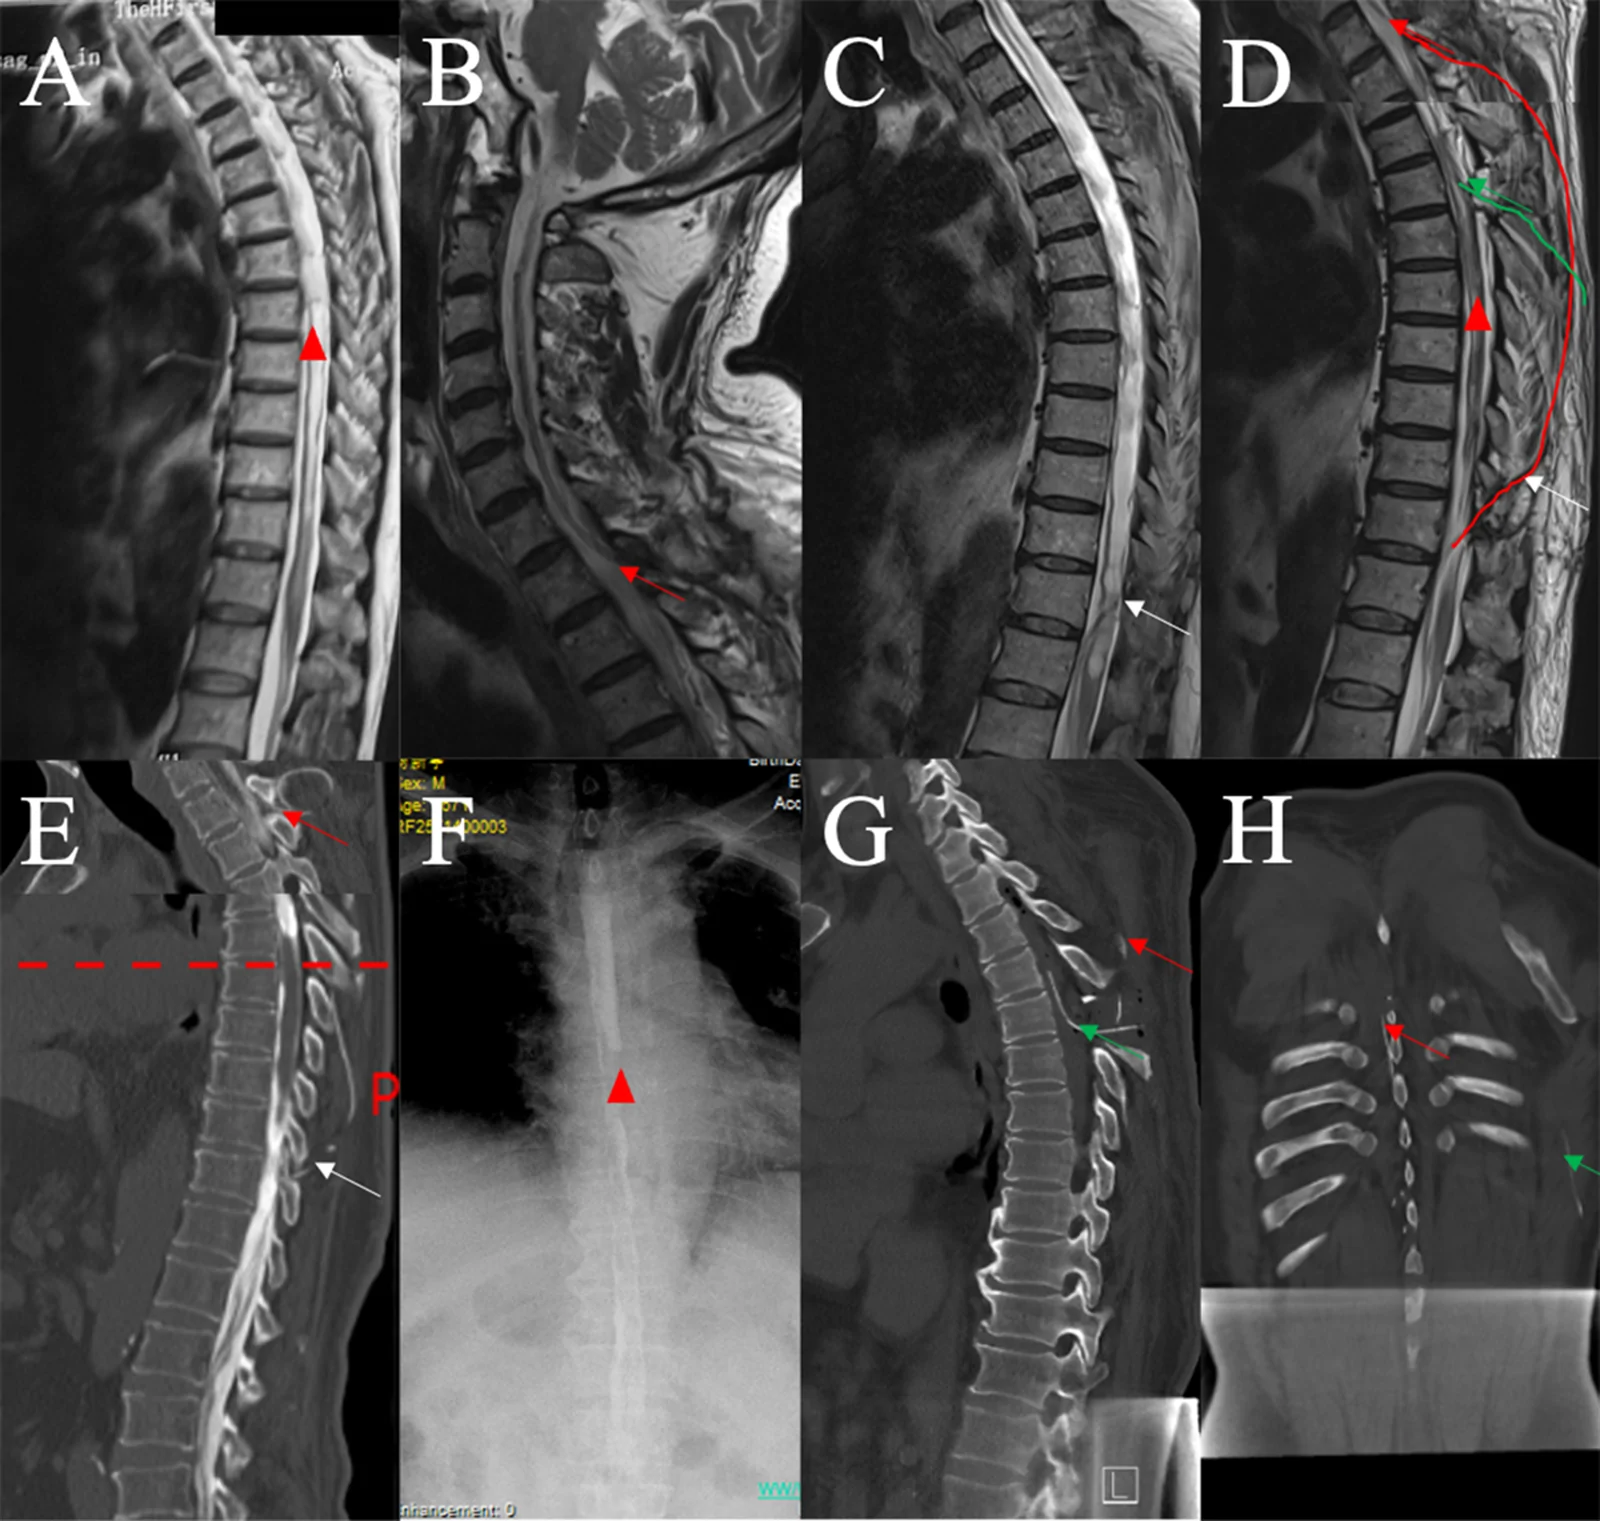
A 60-year-old male, who had undergone an S-S bypass from T2 to T11 two years earlier, presented with progressive lower extremity numbness, weakness, and a one-year history of gait instability. CASE4: (A) Preoperative T2-weighted magnetic resonance (MR) image demonstrates a large syrinx(red triangle) extending from T2 to T12. (B, C) Follow-up T2-weighted MR images obtained 2 years after S-S bypass (from T2 to T11, red and white arrows) show a persistent, stable syrinx. (D) A subsequent T2-weighted MR image following the addition of a thecal-peritoneal shunt (green arrow) reveals a significant reduction in syrinx size. (E, F) Postoperative CT myelography (E) and correlative X-ray (F) after lumbar contrast injection demonstrate patent, unobstructed contrast flow within the spinal canal following the initial S-S bypass procedure (T2 to T11, red and white arrows). (G, H) Postoperative computed tomography (CT) images confirm the final position of both the S-S bypass conduit (from T2 to T11, red and white arrows) and the T4-peritoneal shunt catheter (green arrow)
